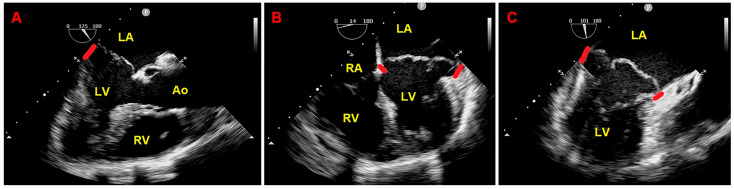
Transesophageal echocardiography. MAD assessment at end-systole from the mid-esophageal three-chamber view (A), four-chamber view (B), and two-chamber view (C). The bold red line indicates the MAD distance. Ao, aorta; LA; left atrium; LV, left ventricle; RA, right atrium; RV, right ventricle. (A) is reproduced from the ref. [4].
